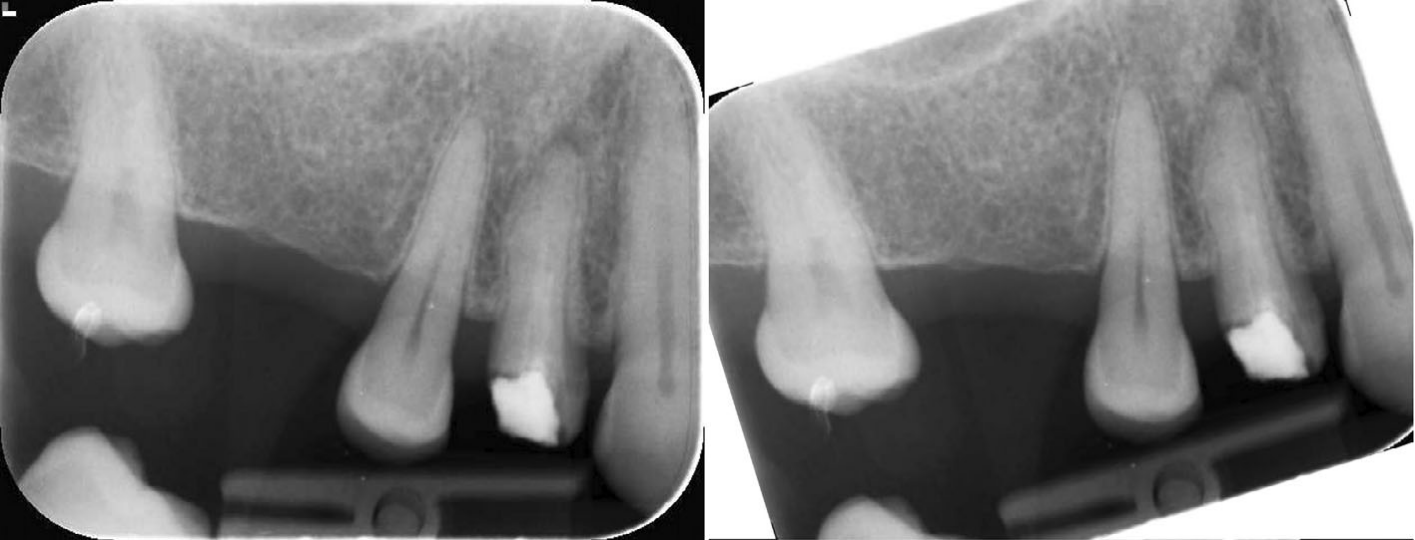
Vertical positioning of the periapical radiograph (second premolar) to be analyzed with Paint 3D.
